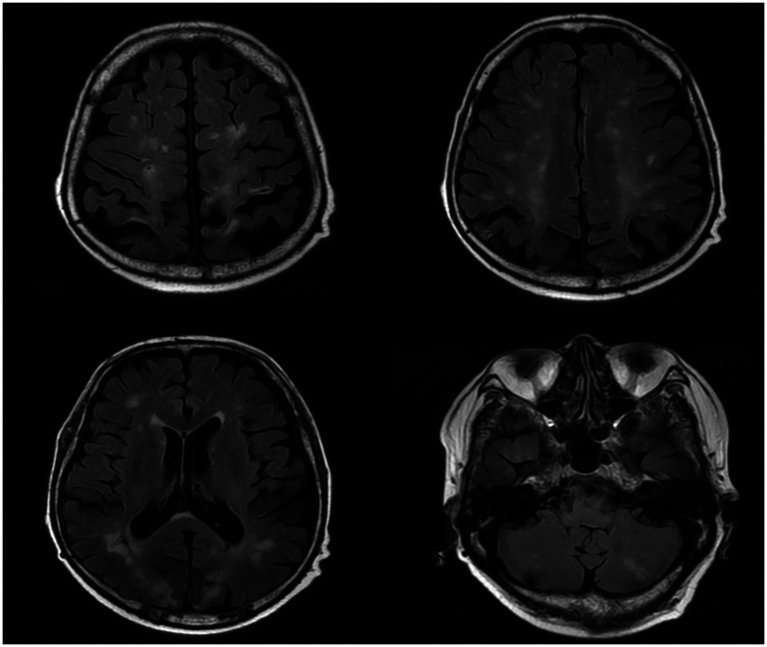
Repeated cranial CT of the patient.
